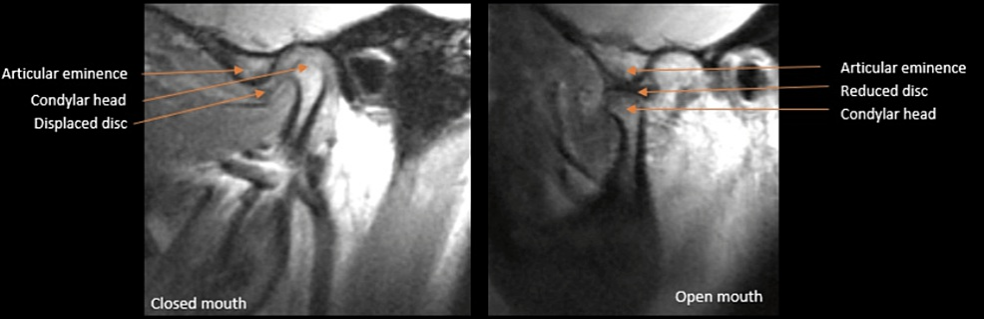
MRI Proton density showing anterior displaced disc on left side closed mouth view and reduced disc over condylar head on open mouth view
